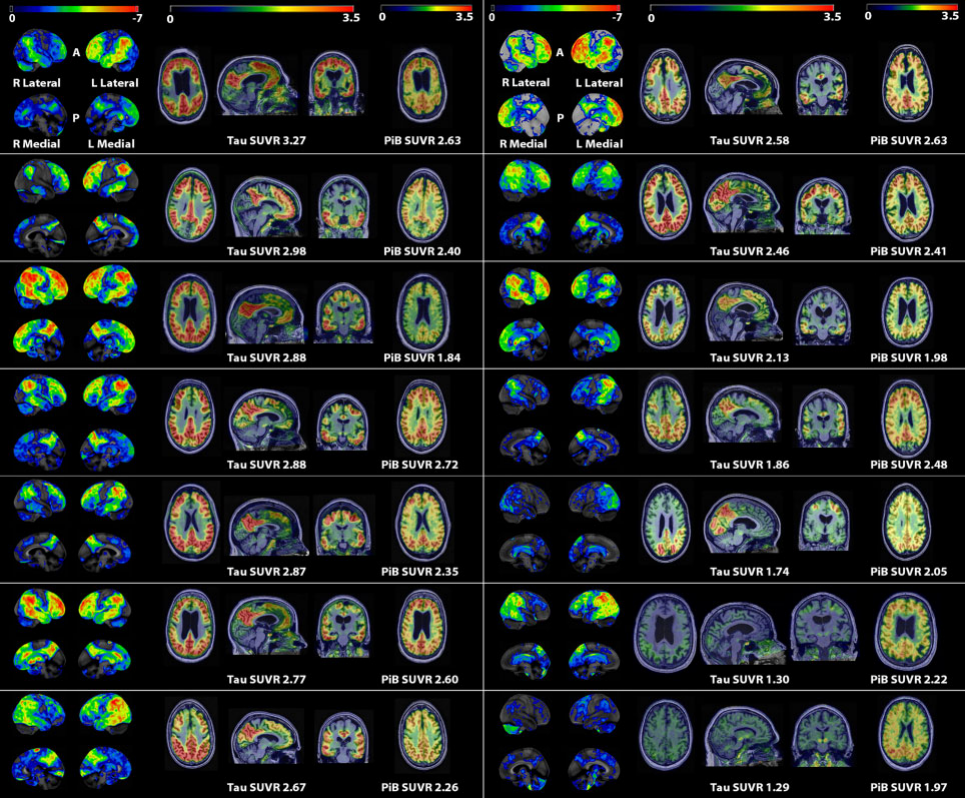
Molecular neuroimaging and heterogeneity in dysexecutive Alzheimer’s disease of the remaining participants with all three molecular imaging modalities available (participant in Fig. 5 not included). Participants are separated into two columns. Within each column: *Left:* FDG–PET (using Cortex ID, GE Healthcare) with z-score bar at the top. *Middle:*^18^F-AV1451 tau PET with SUVR bar at the top and individual meta-ROI SUVR below each image. *Right:* PiB amyloid-PET with SUVR bar at the top and individual meta-ROI SUVR below each image. There is hypometabolism and tau PET uptake predominantly in the dorsolateral prefrontal, lateral temporoparietal, precuneus and posterior cingulate regions, with inter-individual heterogeneity in topography and degree of involvement. CSF p-tau level, tau PET SUVR and mean time lag between CSF and tau PET for each participant can be found in Supplementary Table 1.
